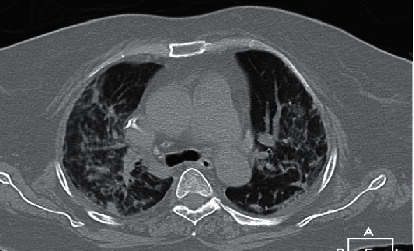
Chest computed tomography on admission showed multilobar ground-glass opacity with reticulation and consolidation in the center and periphery of both lungs.
